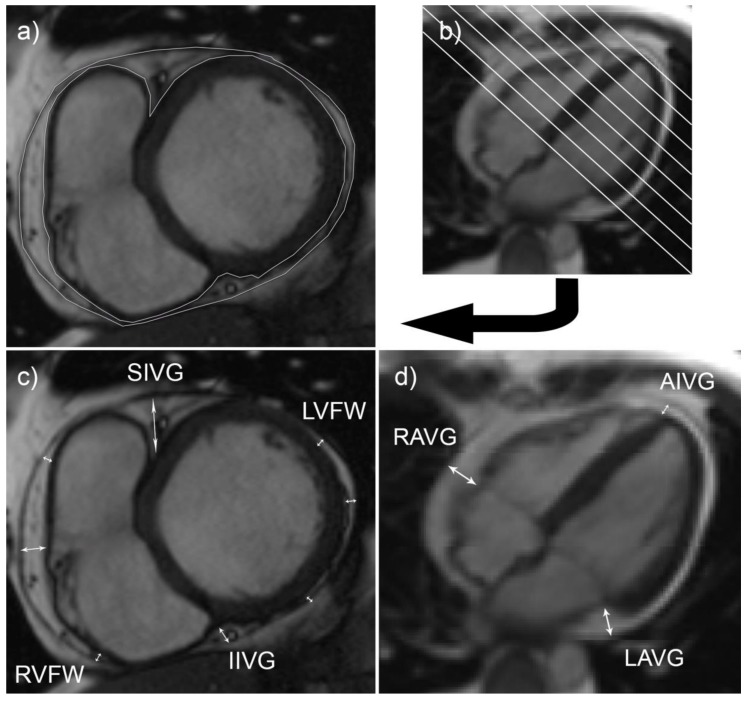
EAT measurements on cardiac magnetic resonance imaging. True fast imaging with steady-state precession (TrueFISP) images of horizontal long-axis (b), (d) and short-axis (a), (c) views in end-diastole were used. (a) and (b) images show measurement of EAT volume: an outlined EAT area (a) in one of the slices (b). (c) and (d) images show measurement of EAT thickness on the right ventricular free wall (RVFW), left ventricular free wall (LVFW), in the superior interventricular groove (SIVG), inferior interventricular groove (IIVG), anterior interventricular groove (AIVG), right atrioventricular groove (RAVG), left atrioventricular groove (LAVG).
